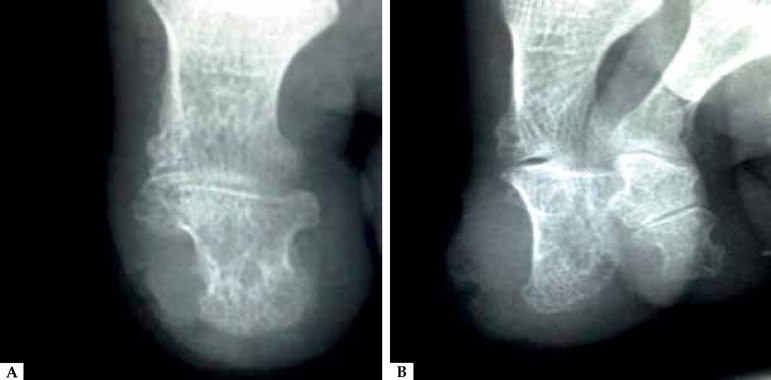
A) AP X-ray: No bone involvement; B) X-ray Profile:
